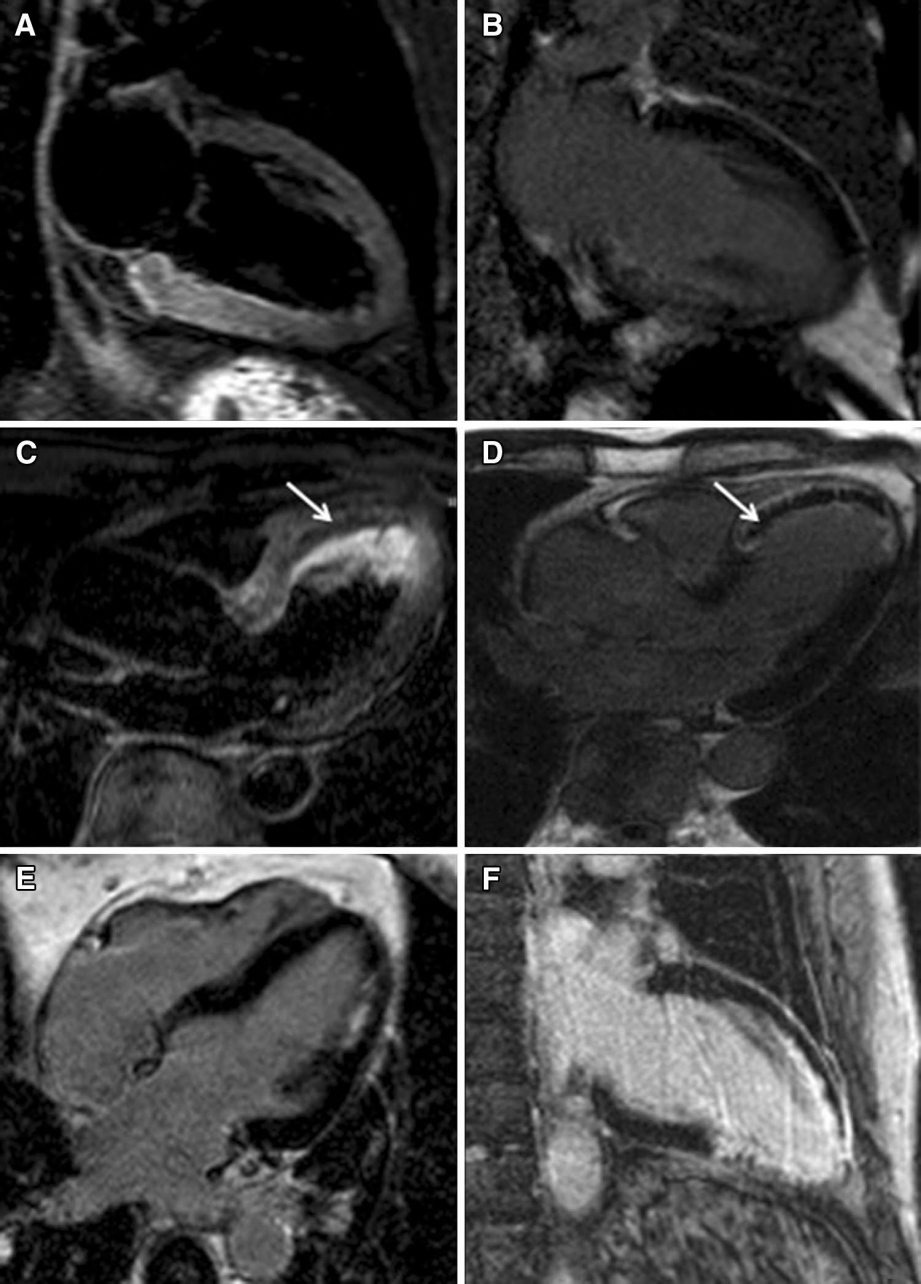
Myocardial infarction: overview. T2-weighted two chamber long axis view showing myocardial oedema in the basal to mid-cavity inferior wall (a) with concomitant subendocardial late gadolinium enhancement (LGE) in the post-contrast sequence (b) in a patient with acute subendocardial infarction in the proximal to mid right coronary artery territory. T2-weighted three chamber long axis view showing myocardial oedema in the mid-apical anteroseptum with evidence of a hypo-intense core (c, *white arrow*), consistent with intramyocardial haemorrhage, in a patient with transmural infarction in the distal left anterior descending territory (d) with persistence of microvascular obstruction (d, *white arrow*). Four chamber long axis post-contrast view showing subendocardial LGE of the mid-cavity anterolateral wall (e). Two chamber long axis post-contrast view showing transmural LGE of the mid-apical inferior wall (f)
